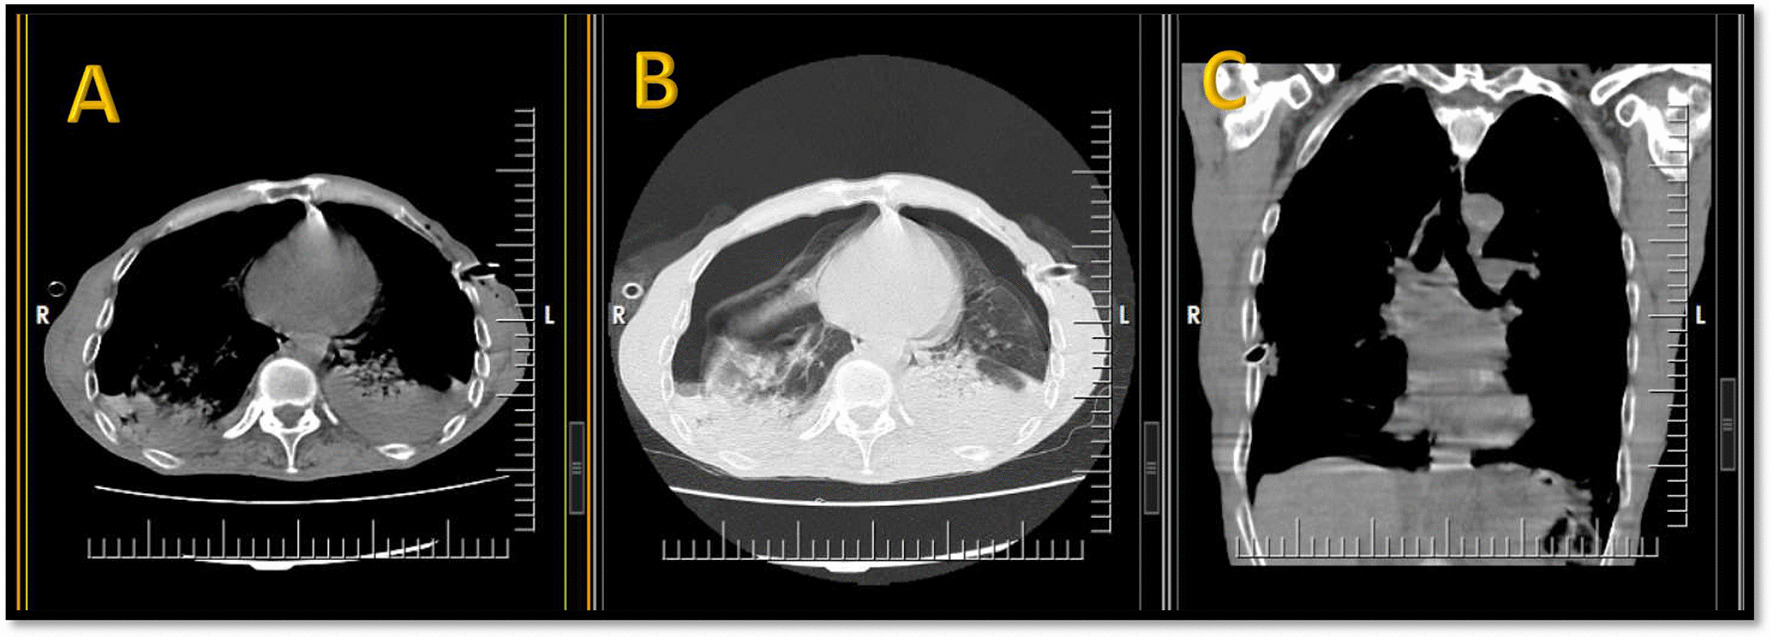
Spiral chest CT scan without contrast (1st admission), inserted bilateral chest tubes, a mediastinal axial view, b lung window axial view, c mediastinal coronal view
